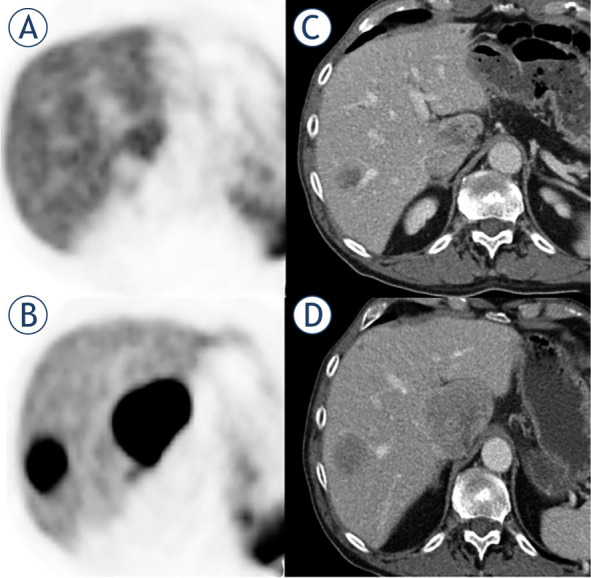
72-year-old male with responding liver metastases of pancreatic neuroendocrine tumor. on the pretherapeutic PET/CT (A, B) there were high tumor-to-liver (t/l) ratios. after three months of treatment with CAPTEM, both liver metastases showed a shrinkage in size, but also a significantly reduced uptake of ^68^ga-dotatate compared to pretherapeutic PET/ CT (C, D). CAPTEM = capecitabine and temozolomide
